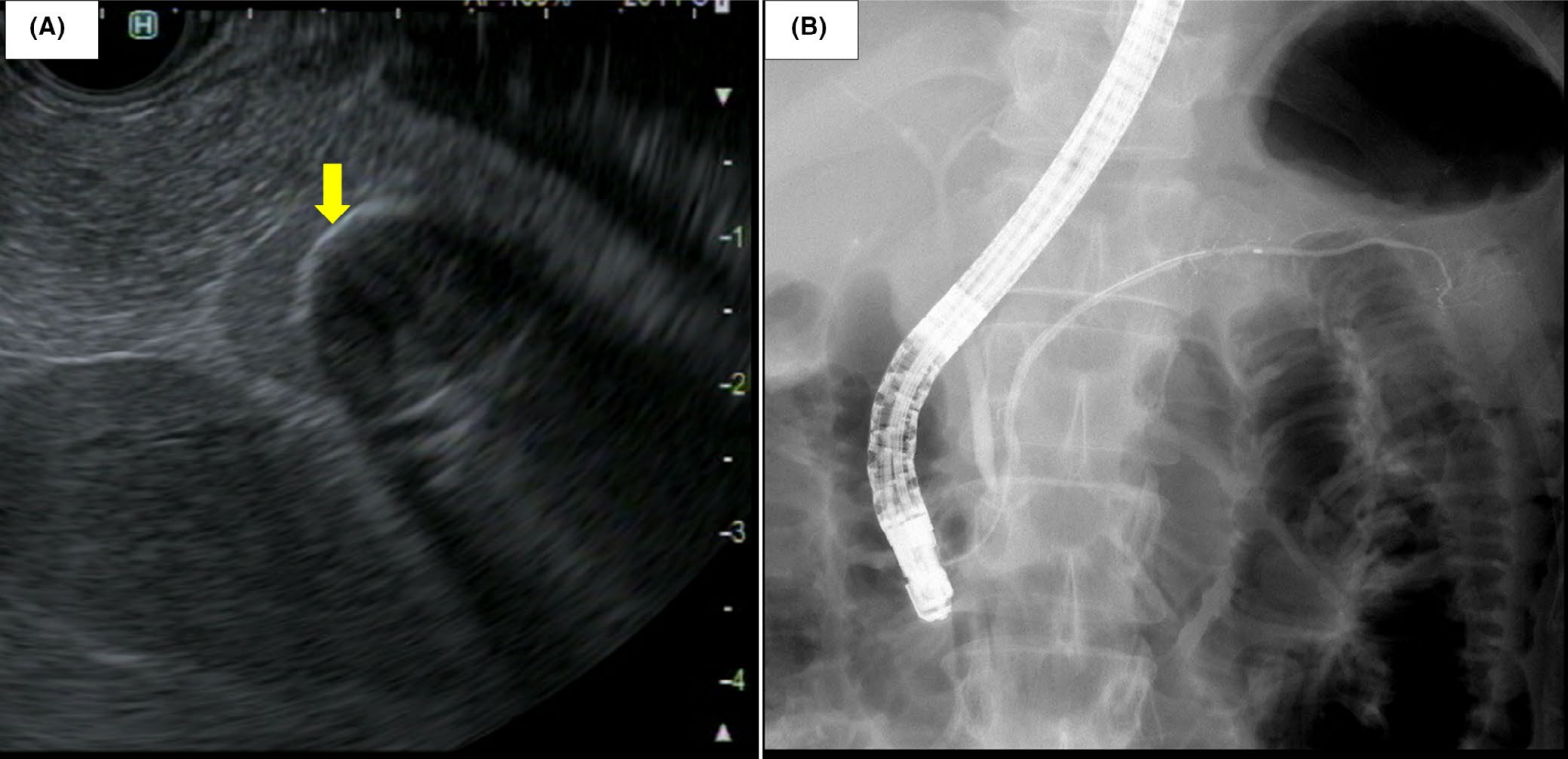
Endoscopic imaging: A, Endoscopic ultrasonography (EUS) shows a cyst with calcification in the margin (arrow). B, Endoscopic retrograde cholangiopancreatography shows a normal main pancreatic duct with no communication with the cystic lesion
